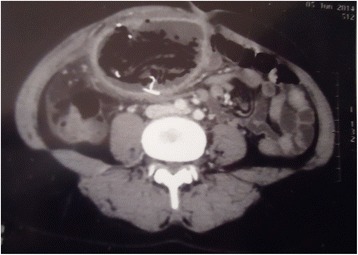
Abdominal CT scan; well limited hypogastric mass (gossypiboma) with hydro aeric content and rares calicifications andhyperdense structure coresponding to a radiopaque marquer
